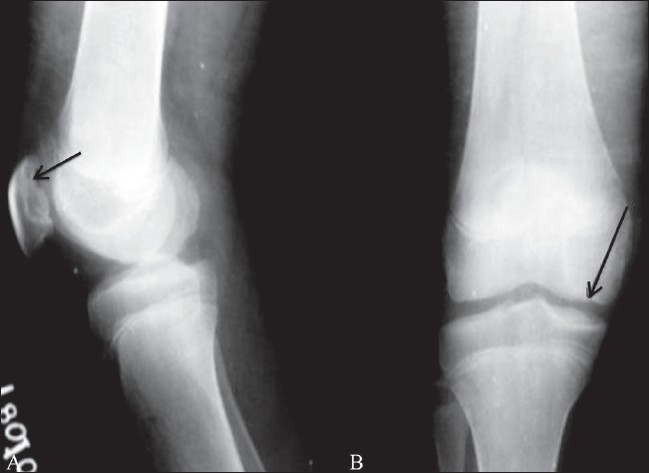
Lateral (A) and anteroposterior (B) radiographs of the left knee show patellar beaking in the upper pole and subchondral irregularity involving the articular surface (black arrow in A). Mild flattening of both the femoral condyles is seen (black arrow in B). However the metaphysis and physeal plate appear normal
